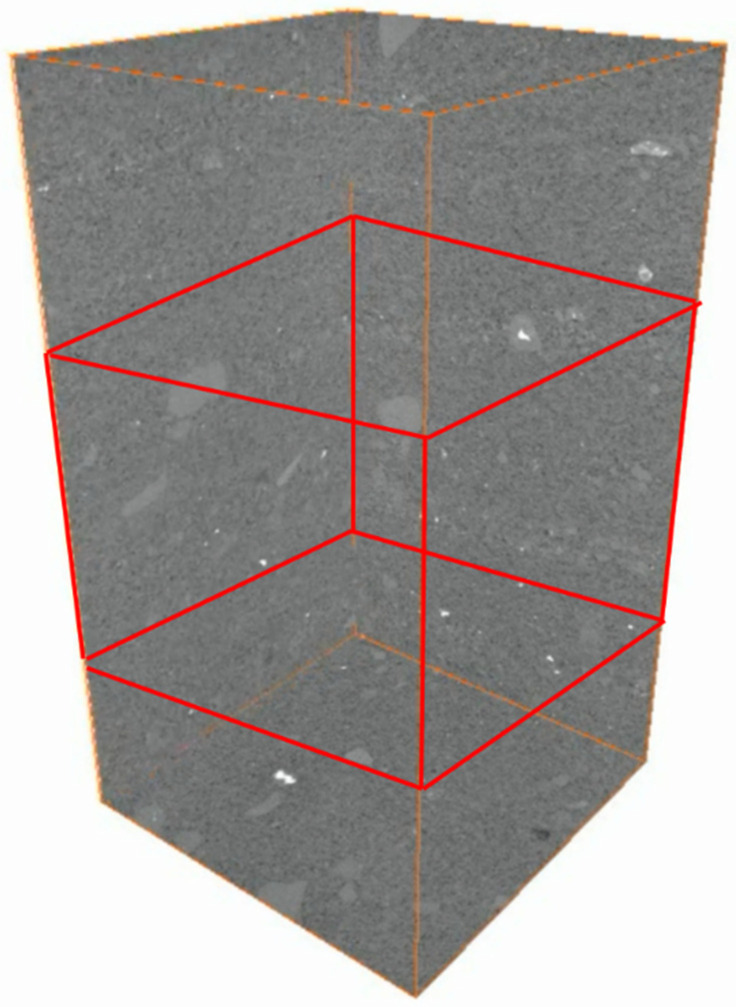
CT scan of rectangular core (resolution 22.5 µm).
